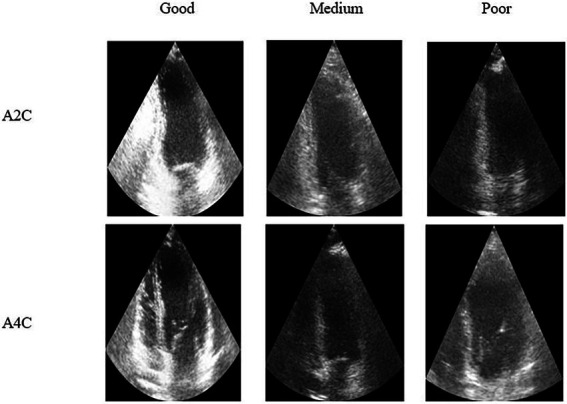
Representative echocardiography images, across two different cardiac views, from good to poor quality ultrasound [images from (12)].
